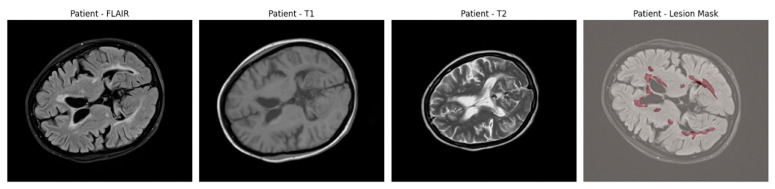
Axial brain slices from a representative MS patient showing the T1-weighted, T2-weighted, and FLAIR MRI sequences. The final panel overlays the expert-annotated lesion segmentation (in red) on the FLAIR image.
